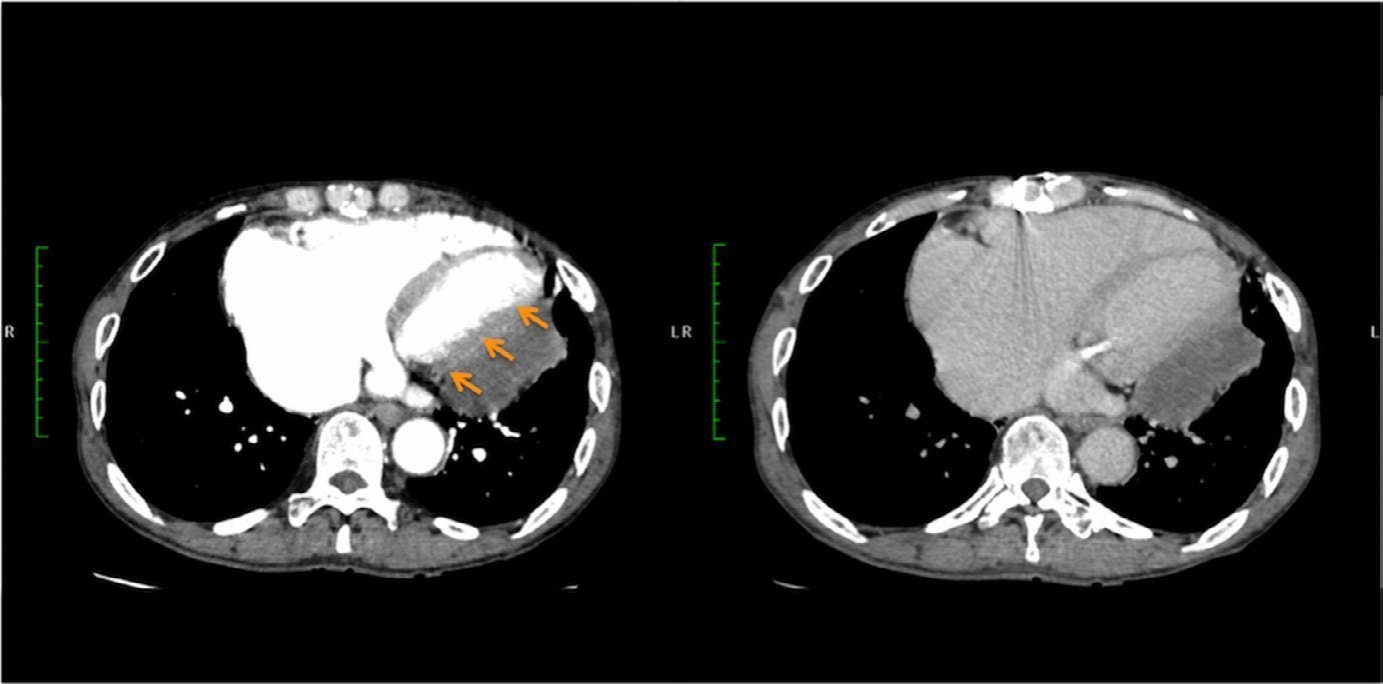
Preoperative contrast‐enhanced computed tomography showing the intrapericardial hematoma compressing the left ventricle. The hematoma was enhanced in the delayed‐phase imaging
